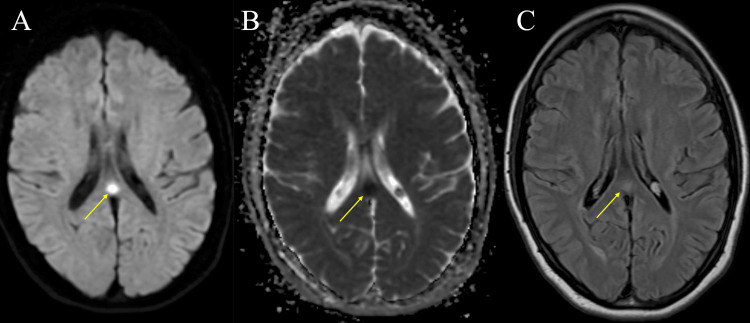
Case 7: MRI findings of CLOCCs (A) DWI shows a high-signal-intensity lesion in the corpus callosum (yellow arrow). (B) The ADC map shows a corresponding low-signal-intensity lesion, indicating restricted diffusion (yellow arrow). (C) FLAIR imaging shows high signal intensity in the corpus callosum (yellow arrow). A follow-up MRI was not available for this case. ADC, apparent diffusion coefficient; CLOCCs, cytotoxic lesions of the corpus callosum; DWI, diffusion-weighted imaging; FLAIR, fluid-attenuated inversion recovery
